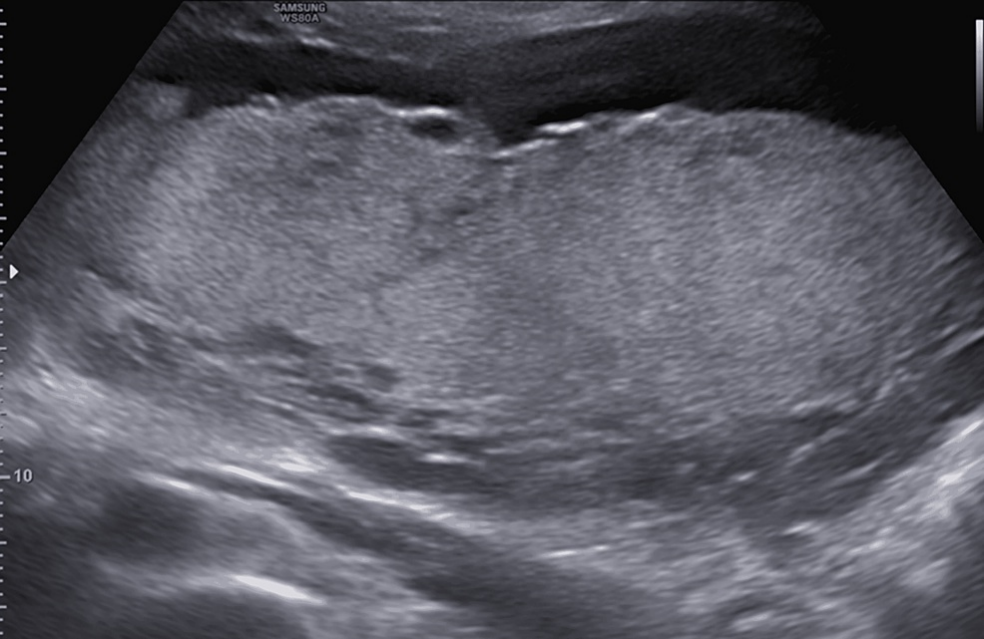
Ultrasonographic placental image at 32nd week of gestation: The pathological placental findings have been resolved.
